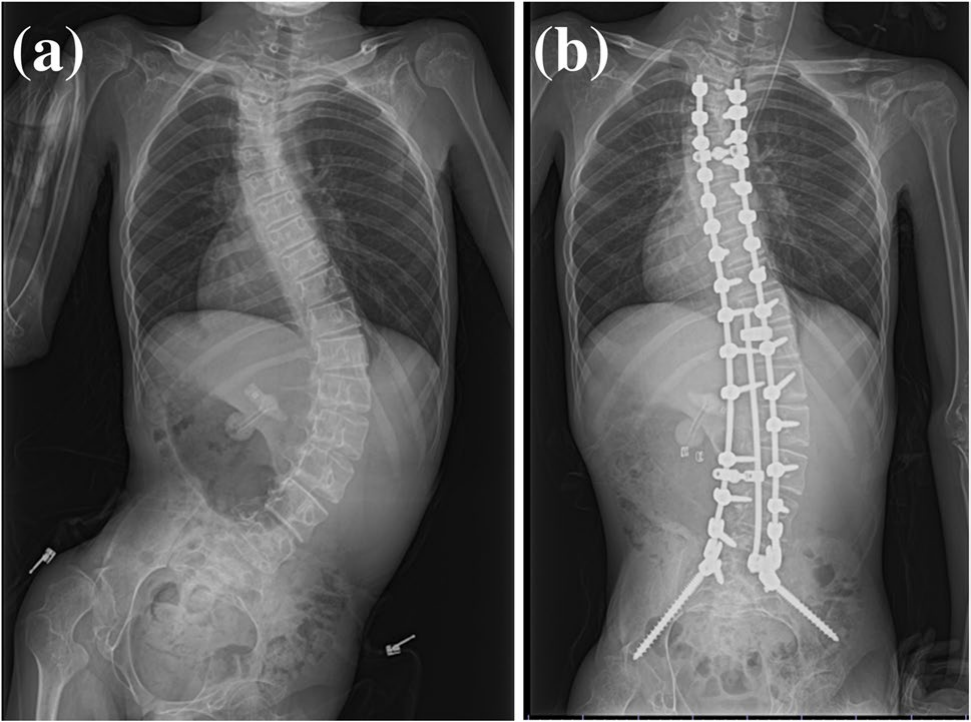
Preoperative and postoperative chest and abdominal radiographs images. (a) Preoperative radiograph showing severe neuromuscular scoliosis with marked thoracic deformity and reduced thoracic volume. (b) Postoperative radiograph demonstrating correction of spinal alignment after posterior spinal fusion from T3 to the pelvis. The patient presented with severe three-dimensional spinal deformity, with a Cobb angle of 76° and pelvic tilt of 38°. Compression of the lungs was also observed due to thoracic deformity associated with scoliosis
